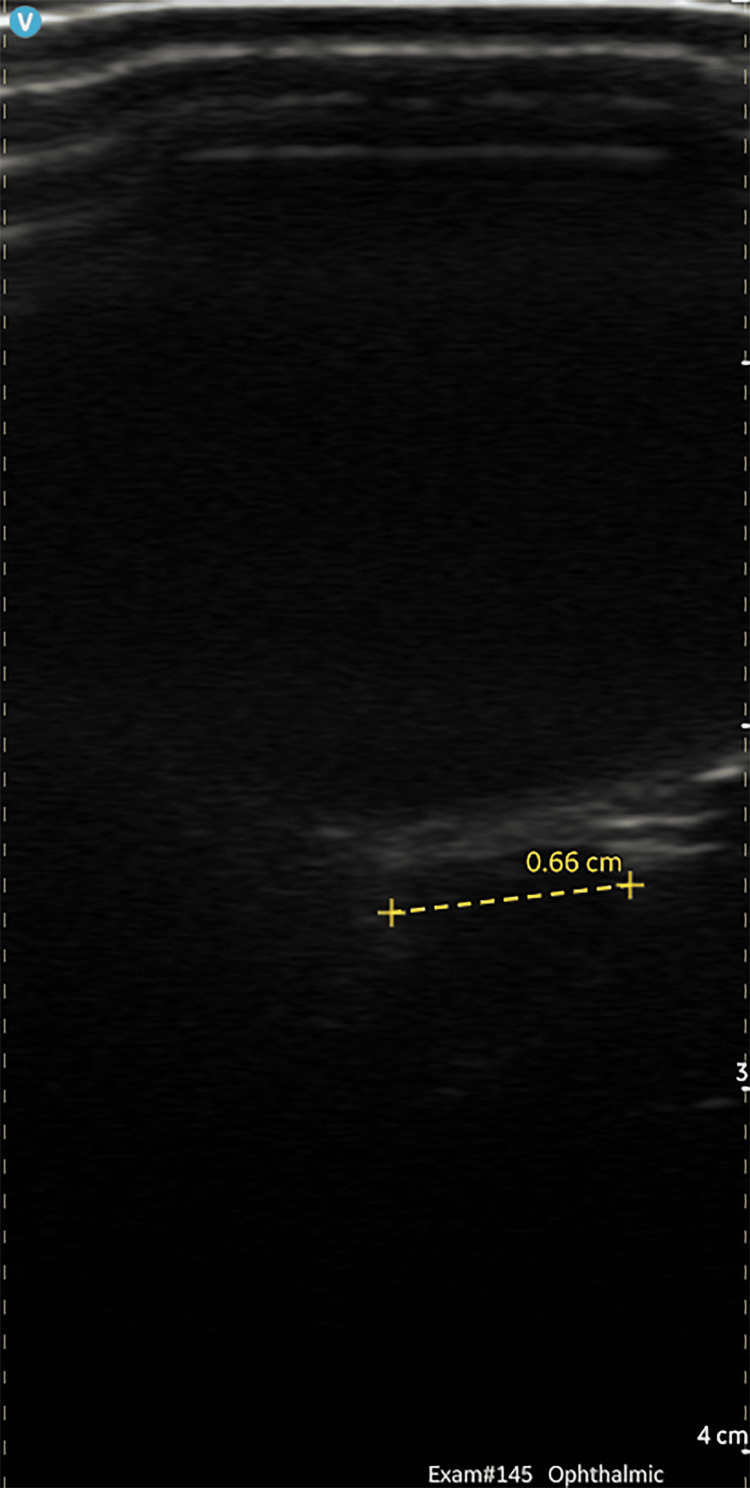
Ocular ultrasound of the right eye demonstrating an increased optic nerve sheath diameter (ONSD) measuring 6.6 mm. An ONSD greater than 5.0–5.7 mm is suggestive of raised intracranial pressure.
